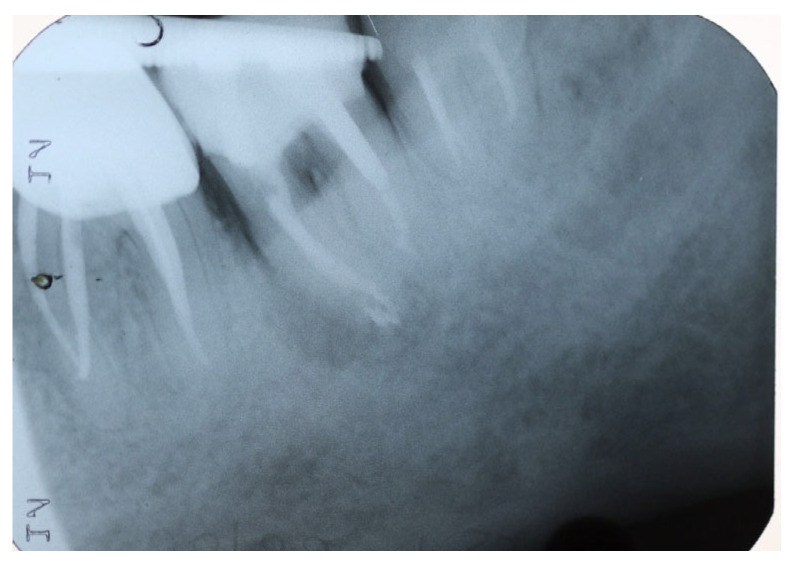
Periapical X-ray of tooth #37 showing a J-shaped radiolucent area at the mesial root.
